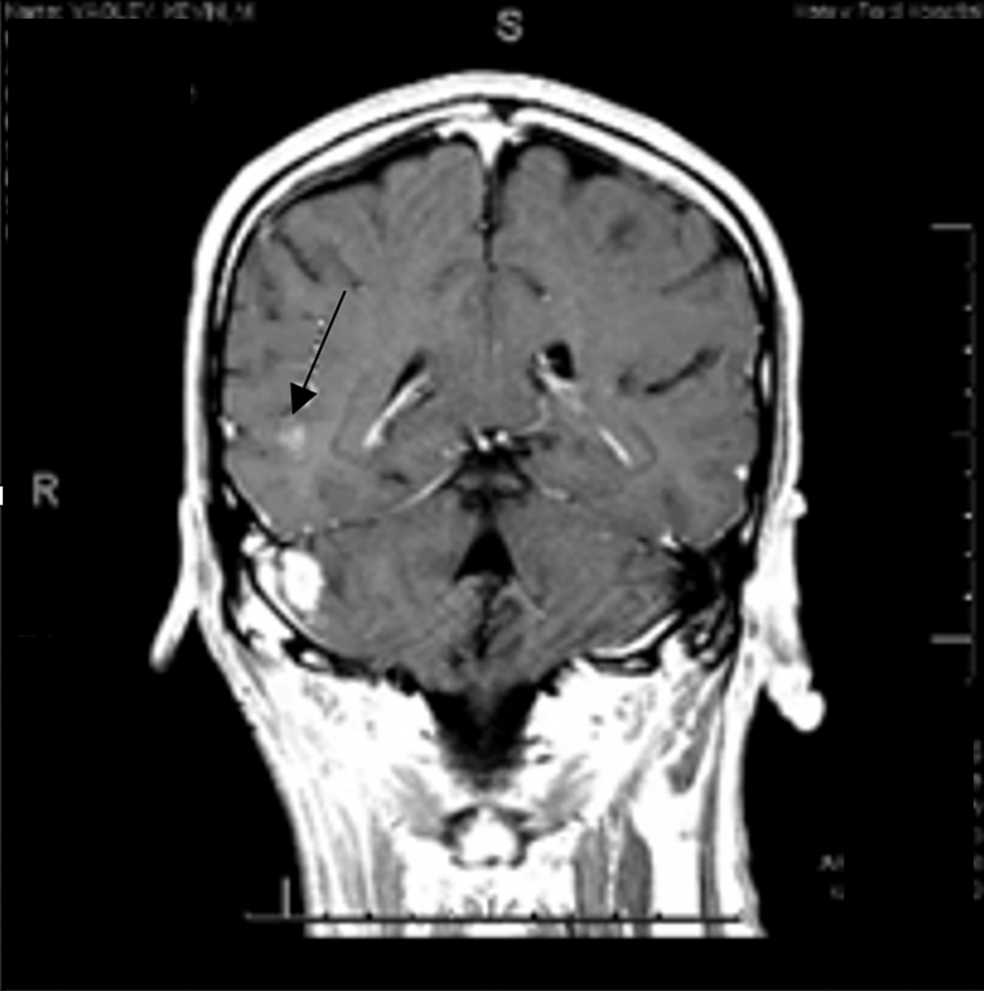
MRI with initial concerns of metastasis vs. radiation induced changes There are new and increasing areas of restricted diffusion within the subcortical white matter of the right temporal lobe. These findings were initially described as nonspecific and differential considerations included cerebellitis/cerebritis, radiation injury, and metastatic disease.
